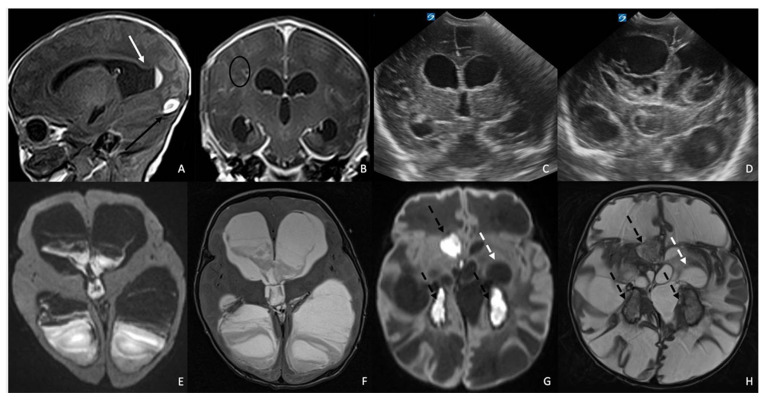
Serial brain MRIs performed at 10 days (A,B), 2 months and 1 week (E,F), and 4 months of age (G,H), and serial brain USs performed at 1 (C) and 2 (D) months of age in a boy diagnosed with *Listeria monocytogenes* infection. Baseline pre-contrast T1WI shows ventricular dilatation, intraventricular hemorrhage (white arrow in (A)), and thrombosis of the right transverse sinus (black arrow in (A)). Post-contrast T1WI confirms ventricular dilatation and demonstrates linear enhancement of the ventricular walls, consistent with ventriculitis, as well as meningeal enhancement consistent with meningitis. Focal hypointense lesions with peripheral enhancement are evident in the right parietal periventricular tissue, consistent with parenchymal abscesses (black oval in (B)). Coronal US images demonstrate initial ventricular dilatation (C), progressing to further enlargement of the ventricular system filled with inhomogeneously hyperechoic material (D). MRI at 2 months and 1 week includes axial DWI (E) and T2WI (F), showing increased ventricular dilation and purulent material within the ventricular system, appearing as inhomogeneous areas of diffusion restriction on DWI (E) and irregular foci of hypointensity within the T2-hyperintense ventricles (F). Follow-up MRI at 4 months includes axial DWI (G) and T2WI (H), demonstrating a marked decrease in ventricular size, with septated ventricles containing residual purulent material that shows intense diffusion restriction on DWI and irregular areas of ventricular hypointensity on T2WI (dotted black arrows in (G,H)), associated with diffuse white-matter hyperintensity and cystic degeneration (dotted white arrows in (G,H)).
